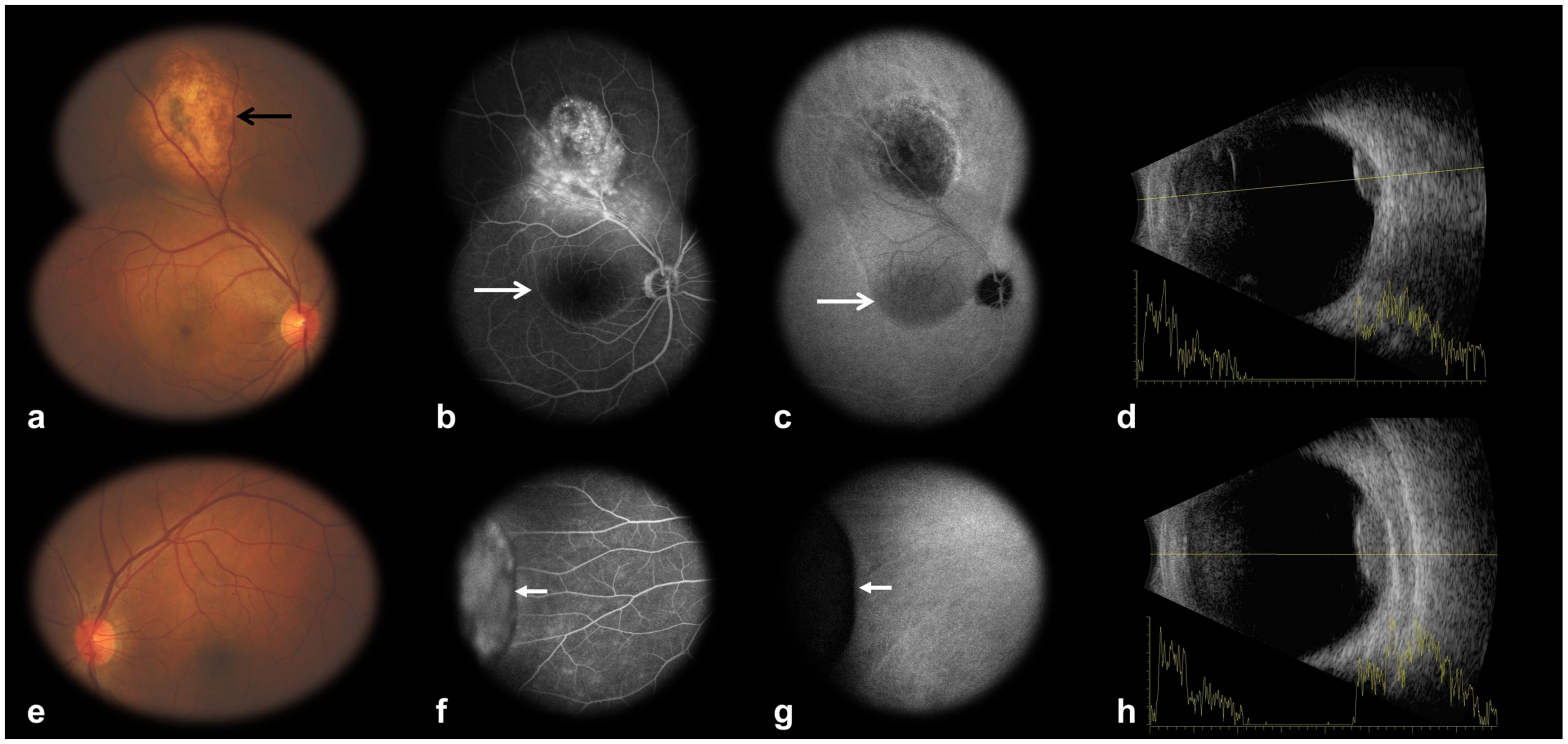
Multiple ophthalmic imaging of bilateral uveal masses. (a) Non-mydriatic fundus photography reveals a subretinal mass in the temporal-superior quadrant of the right eye (black arrow). (b,c) FA demonstrates hyperfluorescence of the right eye mass with late-phase leakage, while ICGA shows hypofluorescence. Note associated subretinal fluid in the macula (white long arrow). (d) B-scan ultrasonography depicts an oval, posteriorly attached mass in the right eye. (e) Fundus photography of the left eye (non-panoramic) failed to capture the ciliary body mass due to its extreme peripheral location. (f,g) FA and ICGA of the left eye nasal periphery (white short arrow) exhibit hyperfluorescence and hypofluorescence, respectively. (h) B-scan ultrasonography identifies a plateau-shaped mass adherent to the left ciliary body. FA, fluorescein angiography; ICGA, indocyanine green angiography.
